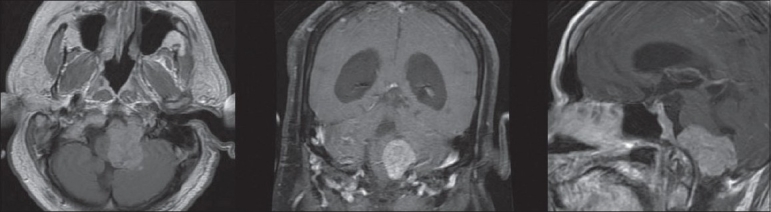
Preoperative MRI of the brain T1-weighted postcontrast infusion sequences in axial, coronal, and sagittal planes demonstrating an extra-axial, eccentrically shaped mass with contrast enhancement in the left aspect of the medullary cistern compressing and displacing the brainstem and cerebellum to the right
